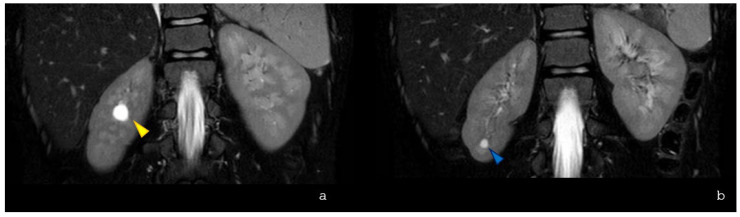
Coronal SPAIR T2-weighted images show the presence of a parapelvic cyst (diameter 11 mm) at the middle of the right kidney (a) [yellow arrowhead] and cortical cyst (diameter 5 mm) at the lower pole of the homolateral kidney (b) [blue arrowhead].
